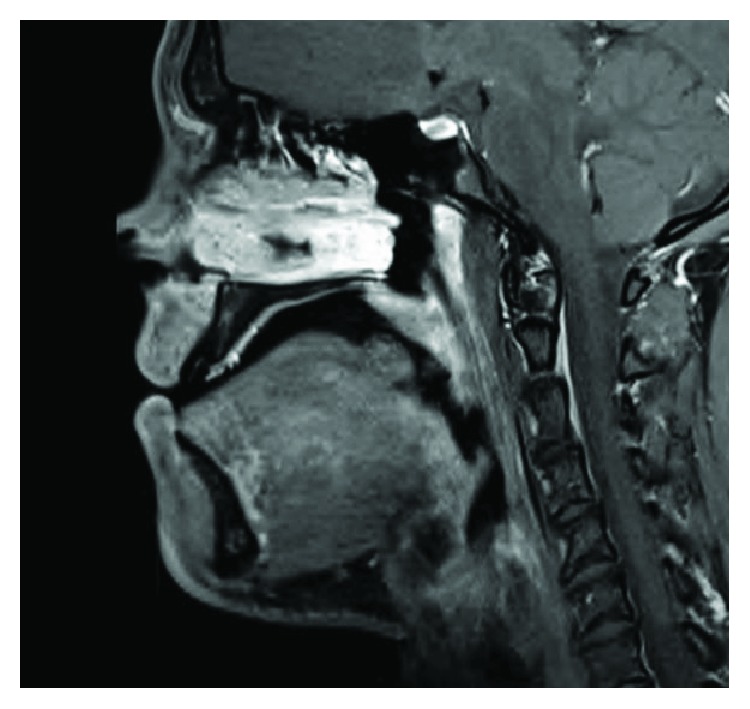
Lingual leiomyomatous hamartoma as seen on magnetic resonance imaging.
